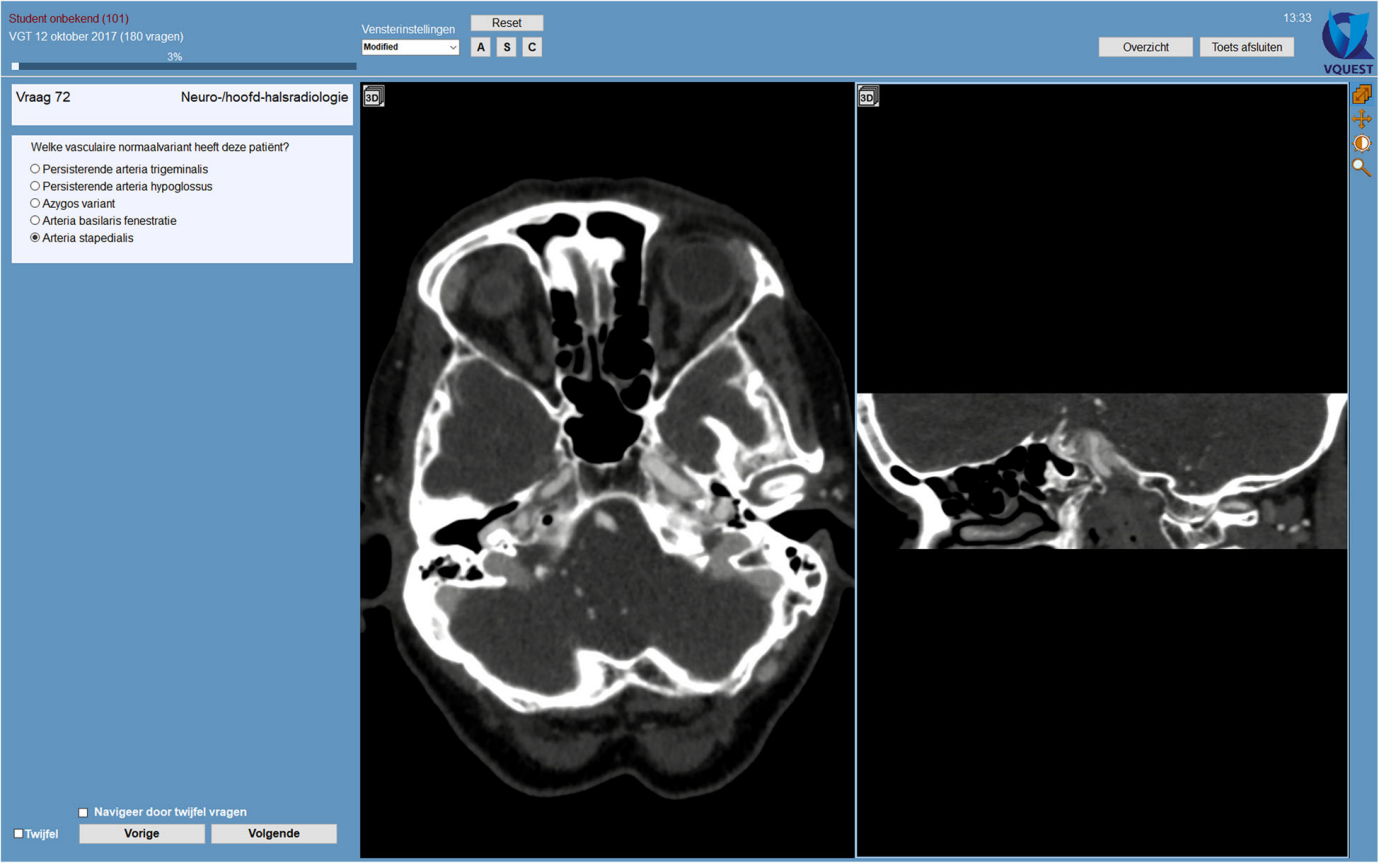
Example of a volumetric image-based test item in the Dutch Radiology Progress Test. It shows an axial volumetric computed tomography scan (left image) with a volumetric reconstruction in the sagittal plane (right image). The participant of the test can scroll through both the axial scan and the sagittal reconstruction for image interpretation. If desired, the participant can also make a reconstruction in the coronal plane (not shown). The item’s question is shown in the left panel in Dutch
